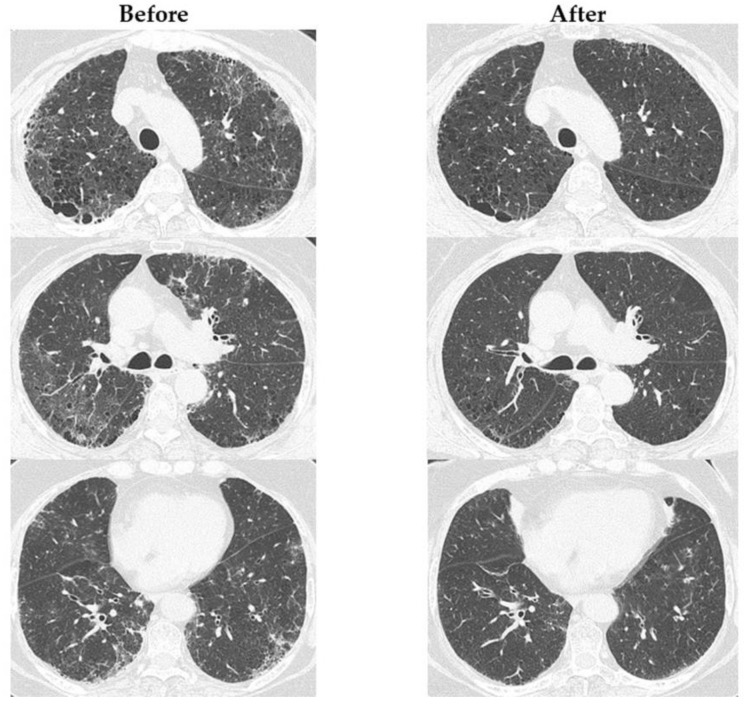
An example case of changes in chest HRCT findings before and 1 year after the start of ABT administration. The images were evaluated at three CT levels: the aortic arch, the left tracheal bifurcation, and 1 cm above the diaphragm. The extent of GGO, septal thickening, and honeycombing in each lung lobe (right upper lobe, middle lobe, lower lobe, left upper lobe, and left lower lobe) was semi-quantitatively assessed in the three images. The total GGO score improved from 11 to 5.33 points, and the total fibrosis score improved from 5.67 to 3.33 points. Before: before ABT administration; After: 1 year after the start of ABT administration; GGO: ground-glass opacity.
